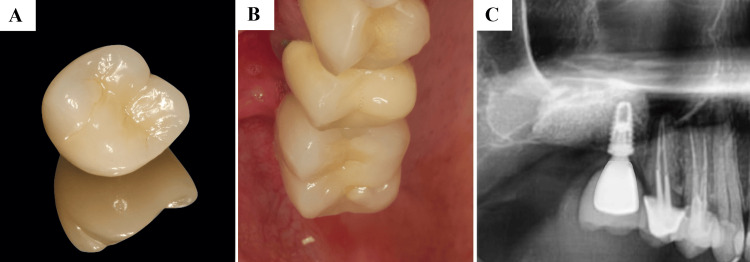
Prosthetic Rehabilitation of the #16 Implant Prosthetic rehabilitation of the #16 implant after the healing period (approximately six months following implant placement). (A)-(C) Clinical and radiographic views showing the definitive crown in function, with adequate peri-implant soft tissue health and proper osseointegration.
